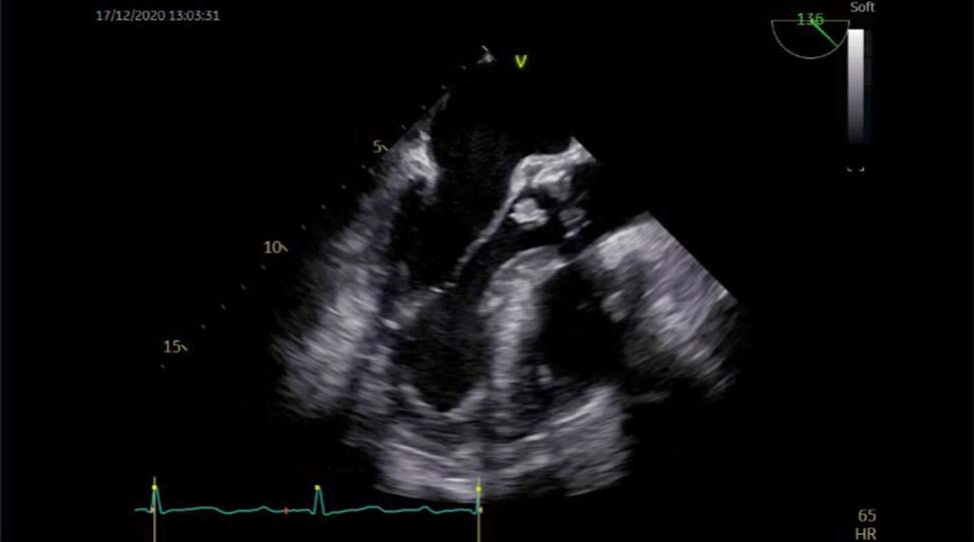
Transthoracic echocardiogram described a mobile hyperechogenic filiform image with 9.2 × 7.5 mm in diameter, in dependence of the right coronary cuspid.
